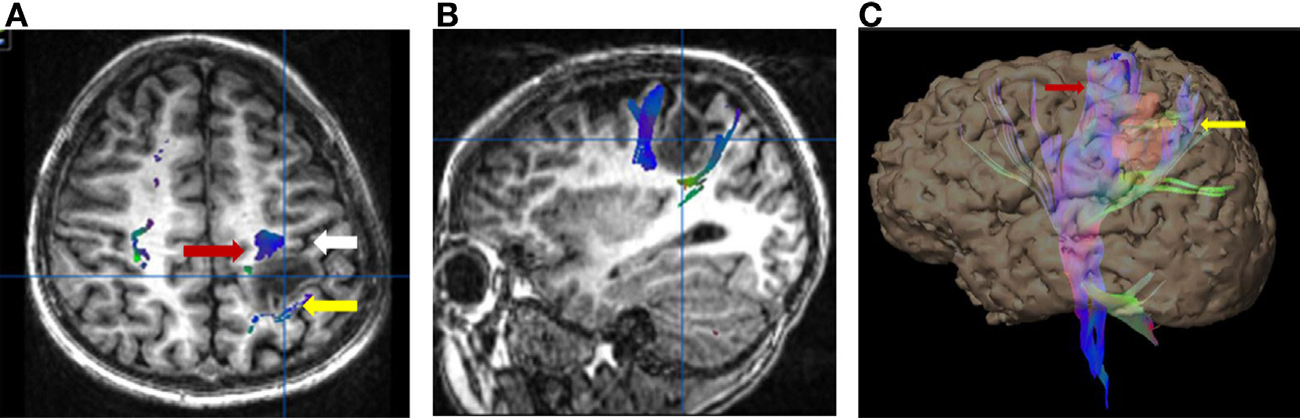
Six-year-old right-handed boy with WHO grade III anaplastic astrocytoma in the subcortical white matter of the left postcentral gyrus. Central sulcus is marked with a white arrow. (A) Axial T1-weighted anatomic image overlaid with directionally color-coded fiber tracking demonstrating the motor (red arrow) and sensory (yellow arrow) corticospinal tracts spread apart by the lesion. (B) Sagittal T1-weighted anatomic image overlaid with directionally color-coded fiber tracking demonstrating the motor (red arrow) and sensory (yellow arrow) corticospinal tracts spread apart by the lesion. (C) Three-dimensional reconstruction with the tumor, colored red, separating the motor (red arrow) and sensory fiber tracts (white arrow). No copyright permissions were required to use these images.
